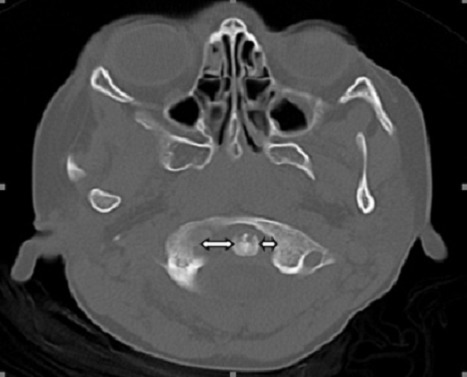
The atlas was rotated on one articular process with 3-5 mm anterior displacement, compatible with type II subluxation in patient's computed tomography
